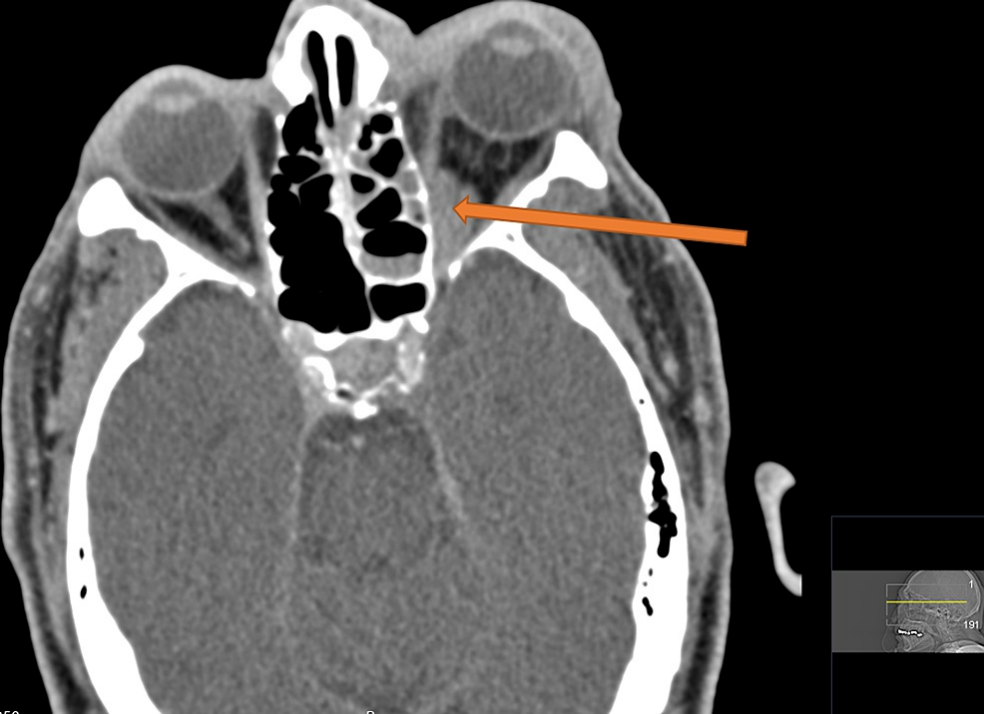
CT of the orbit with contrast. Impression: Left orbital cellulitis likely secondary to extension from sinusitis. There is a 1.7 x 0.7 x 1.4 cm (anteroposterior x transverse x craniocaudal dimensions) hypo-enhancing ill-defined extraconal soft tissue lesion in the posterior inferomedial orbit (orange arrow).
